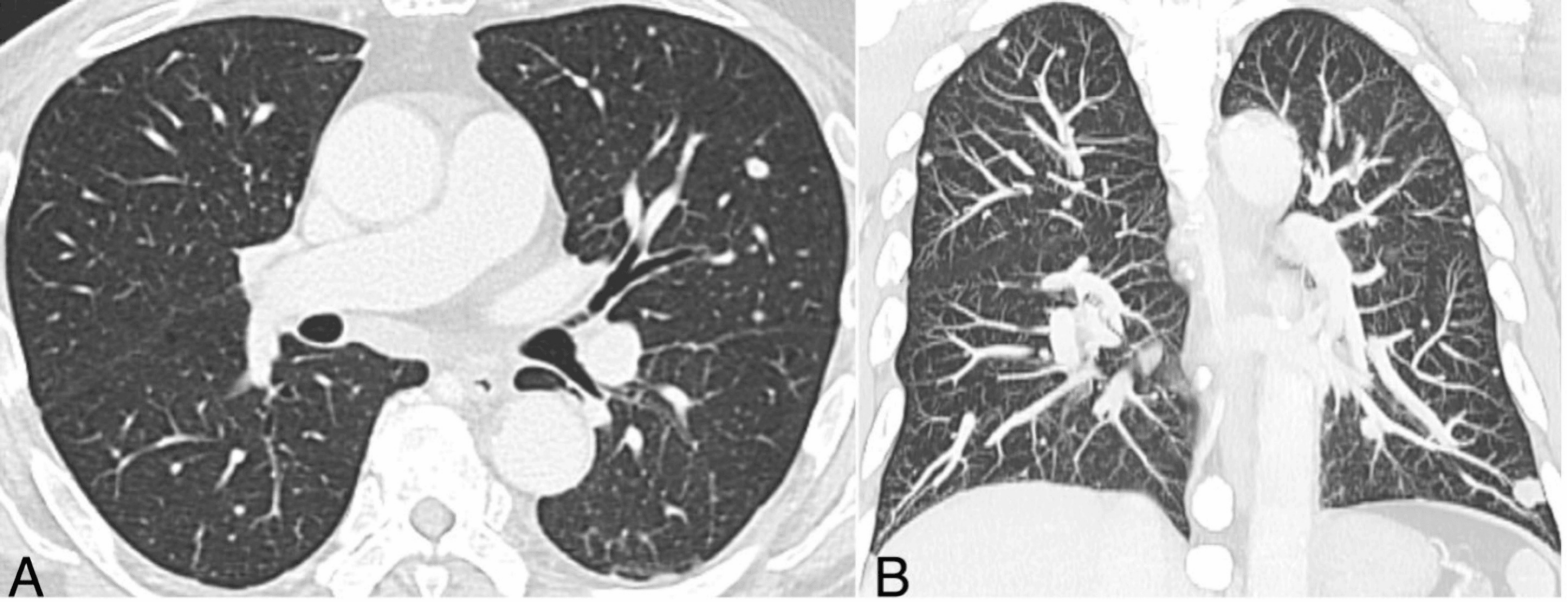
Chest computed tomography demonstrating multiple pulmonary nodules Chest computed tomography images. (A) Axial view and (B) coronal maximum intensity projection image demonstrating multiple randomly distributed pulmonary nodules in both lungs, with interval increases in number and size compared to the previous examination, suggestive of pulmonary metastases.
